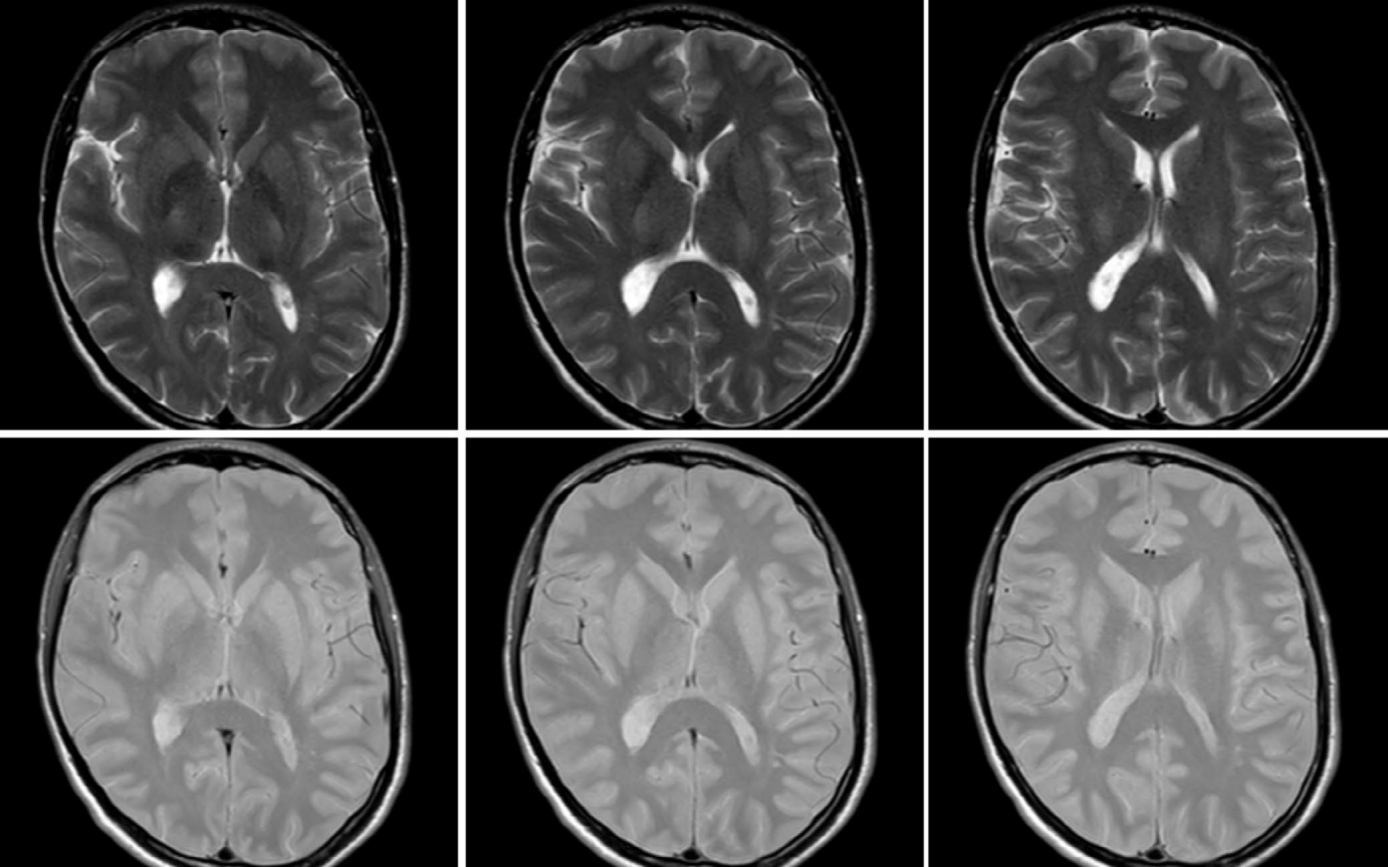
Magnetic resonance imaging of proband performed at the age of 35. Dual‐TSE sequences; images from left to right are arranged in caudocranial order. No signal alterations were signalled except for a blurred hyperintensity of globus pallidus bilaterally.
